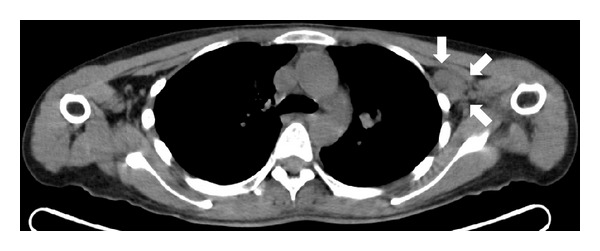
CT image from a 34-year-old female patient diagnosed with cancer in the left breast. The patient had surgery and regular followup care for two years. The followup unenhanced CT of ^18^F-fluoride PET/CT showed multiple enlarged lymph nodes including the left axillary lymph node (arrows, 2.8 × 1.7 cm in size). ^18^F-FDG PET/CT six days later confirmed the presence of these metastatic lymph nodes.
